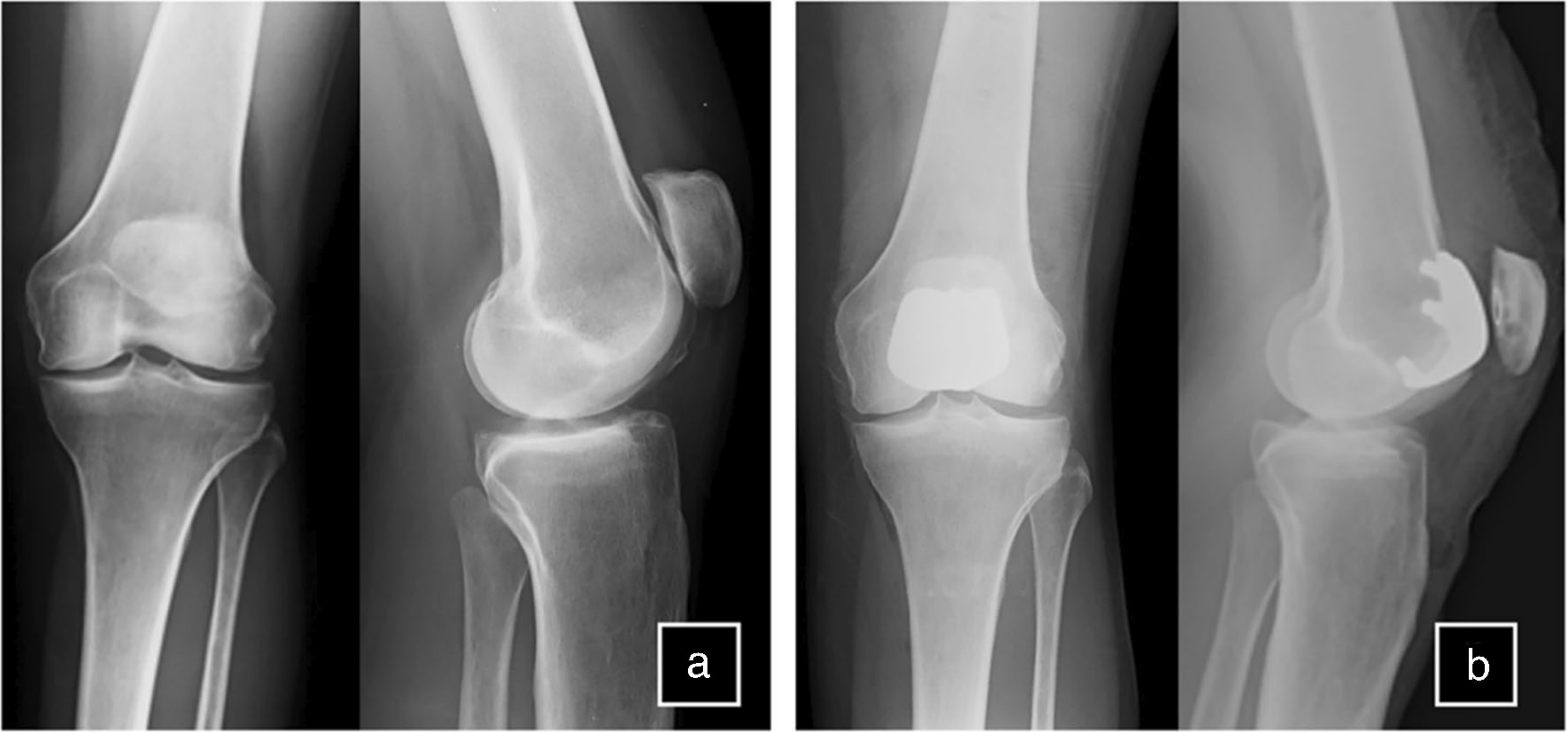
Patellofemoral arthroplasty implantation with imageless robotic-assisted surgical system. Anteroposterior and lateral radiograph of a 61-year-old patient with isolated patellofemoral osteoarthritis (a) before surgery and (b) after surgery
